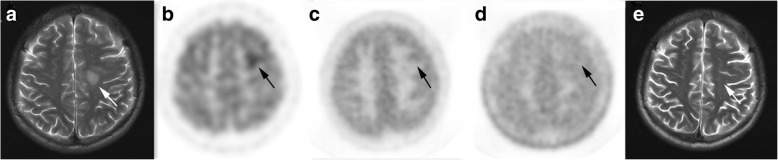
A patient aged 20–25 years with cerebral inflammatory lesion. T2-weighted MRI (a) shows a high signal lesion within the left frontal white matter (*arrow*). The lesion exhibits increased uptake of ^18^F-FDG (b) with equal uptake of ^13^N-NH_3_ (c) and ^11^C-MET (d) compared with contralateral normal brain parenchyma. Repeated MRI (e) shows that the lesion disappeared
